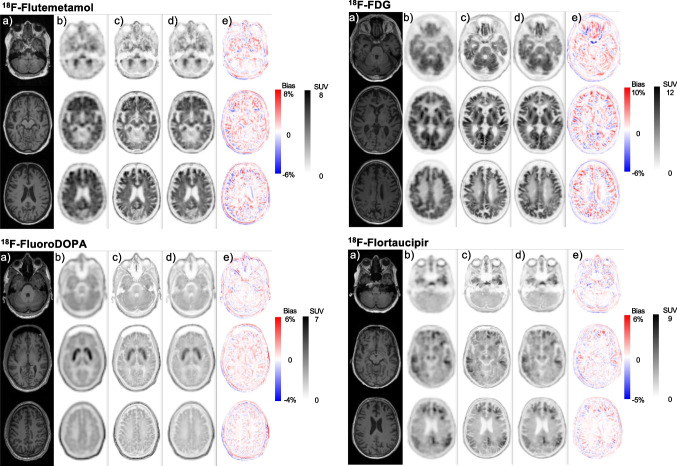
Representative slices of multi-tracer brain PET images of various patients showing (a) coregistered T1-weighted MRI, (b) non-PVC PET images, (c) reference corresponding MRI-guided PVC PET images, (d) DL-predicted PVC PET images, and (e) the corresponding bias maps
